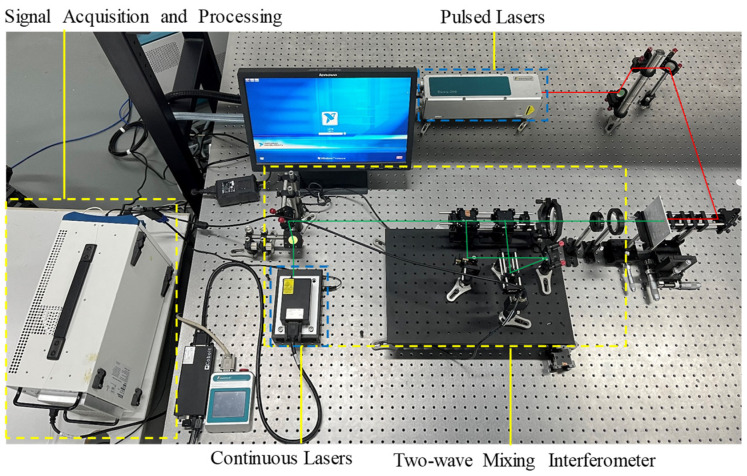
A laser ultrasonic system pulsed laser and an interferometer are used to generate and detect ultrasonic waves.
